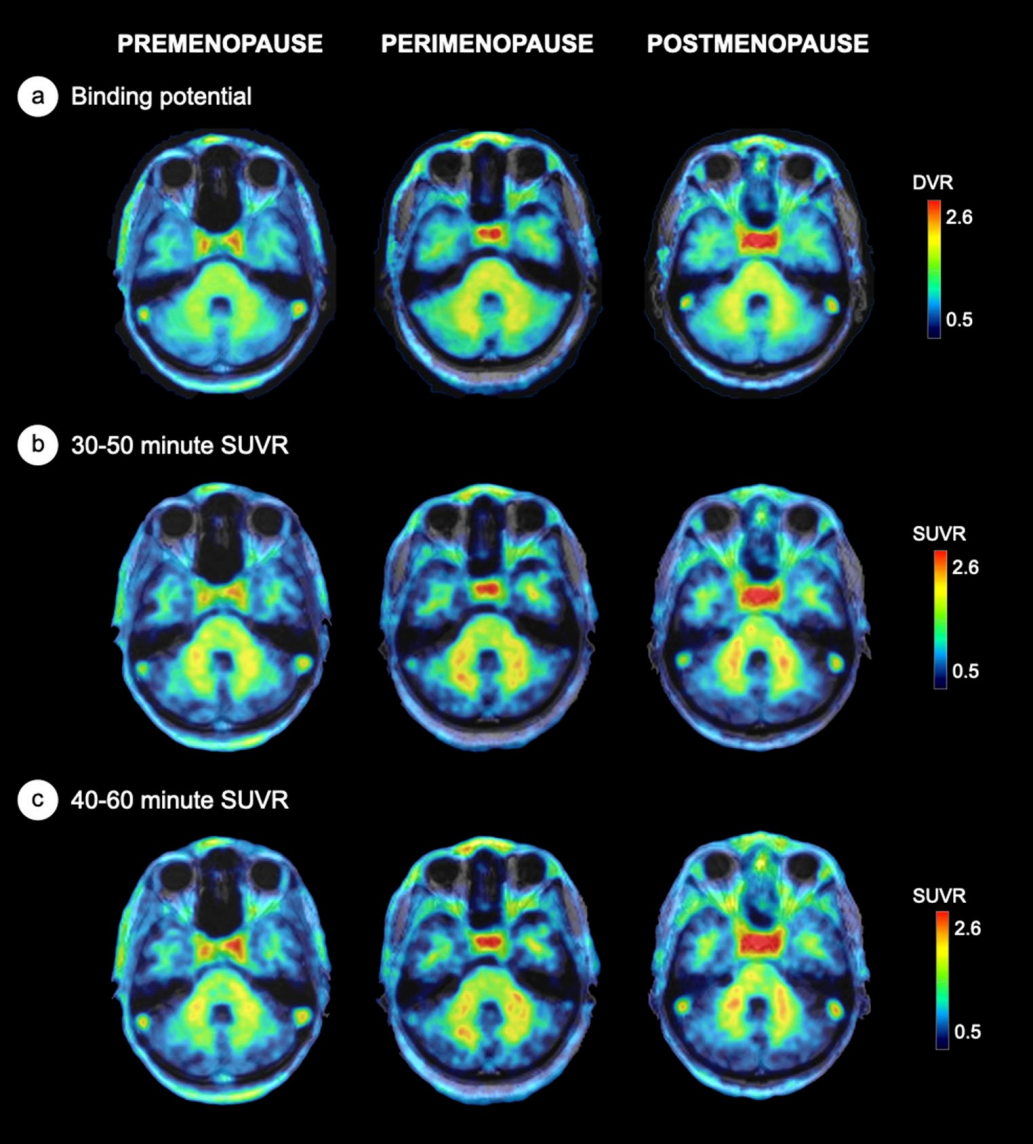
Menopausal stage differences in [¹⁸F]Fluoroestradiol (FES) PET uptake in the pituitary region. Representative parametric [^18^F]Fluoroestradiol (FES) PET images from three participants—premenopausal, perimenopausal, and postmenopausal (left to right)—are shown. ^18^F-FES data are overlaid on coregistered structural MRI: (a) Binding potential (BPnd), used to derive distribution volume ratios (DVR); (b) standardized uptake value ratio (SUVR) from 30 to 50 min post-injection; (c) SUVR from 40 to 60 min post-injection. All methods show a consistent pattern of increased ^18^F-FES signal in the pituitary with advancing menopausal stage.
